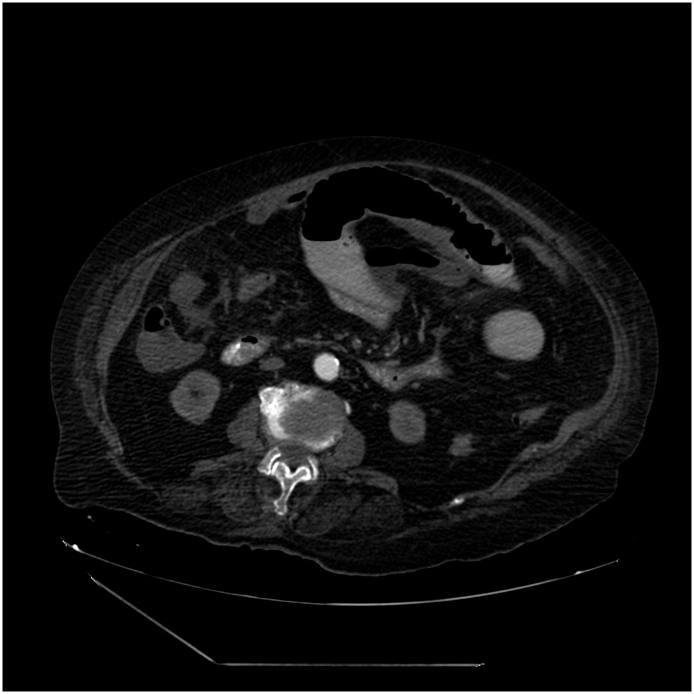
CT-scan showing Early stage of Mesenteric Ischemia with absence of bowel mural enhancement.
